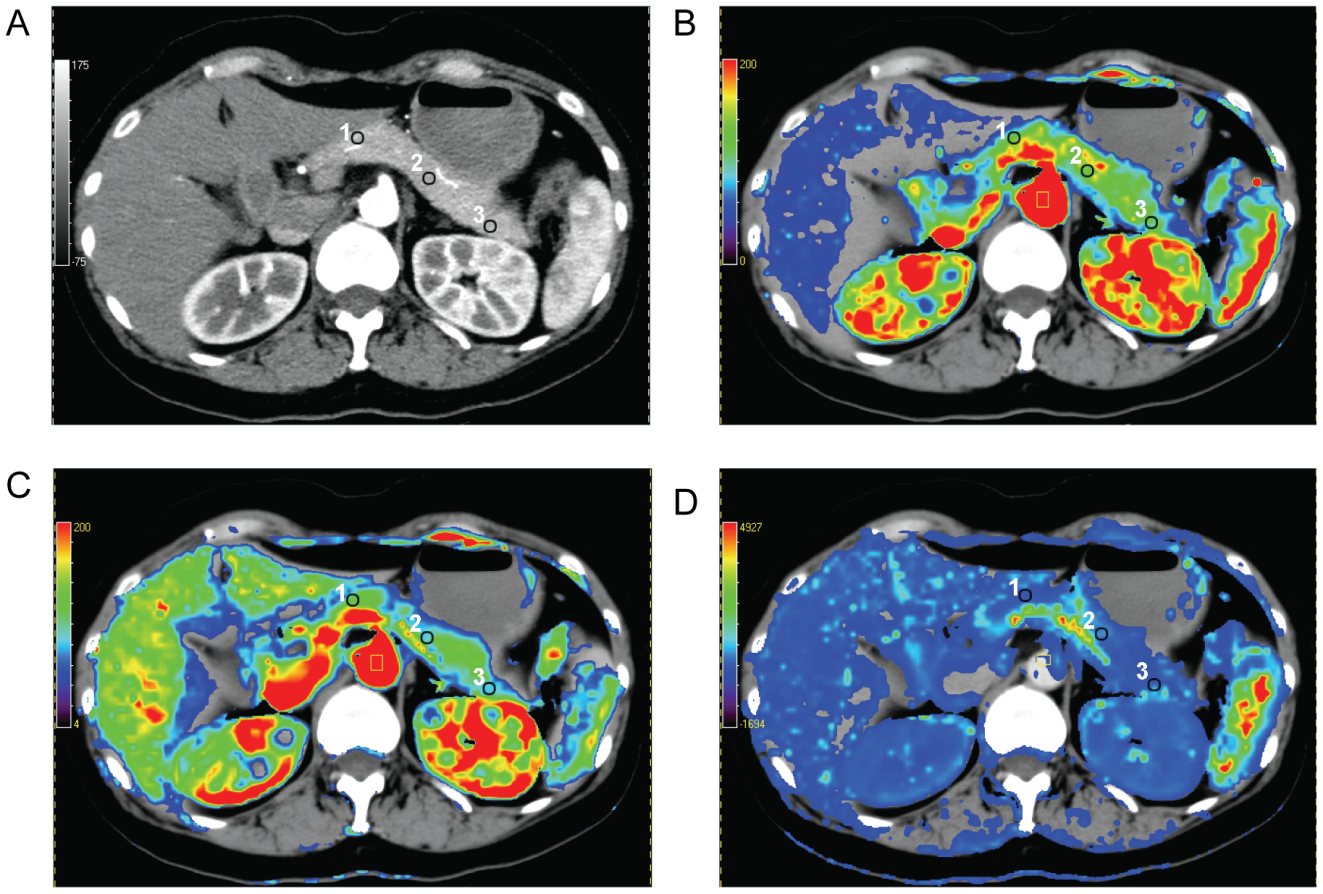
Functional perfusion CT color maps of normal pancreatic tissue in a 48-year-old female. (A) Transverse CT image. (B-D) CT perfusion parametric maps for blood flow, blood volume, and permeability-surface area product. Regions of interest (ROIs) drawn on pancreatic head (ROI 1), body (ROI 2), and tail (ROI 3). For all measurements of the same patient, size, shape, and position of ROIs were kept constant by applying copy-and-paste function at workstations.
